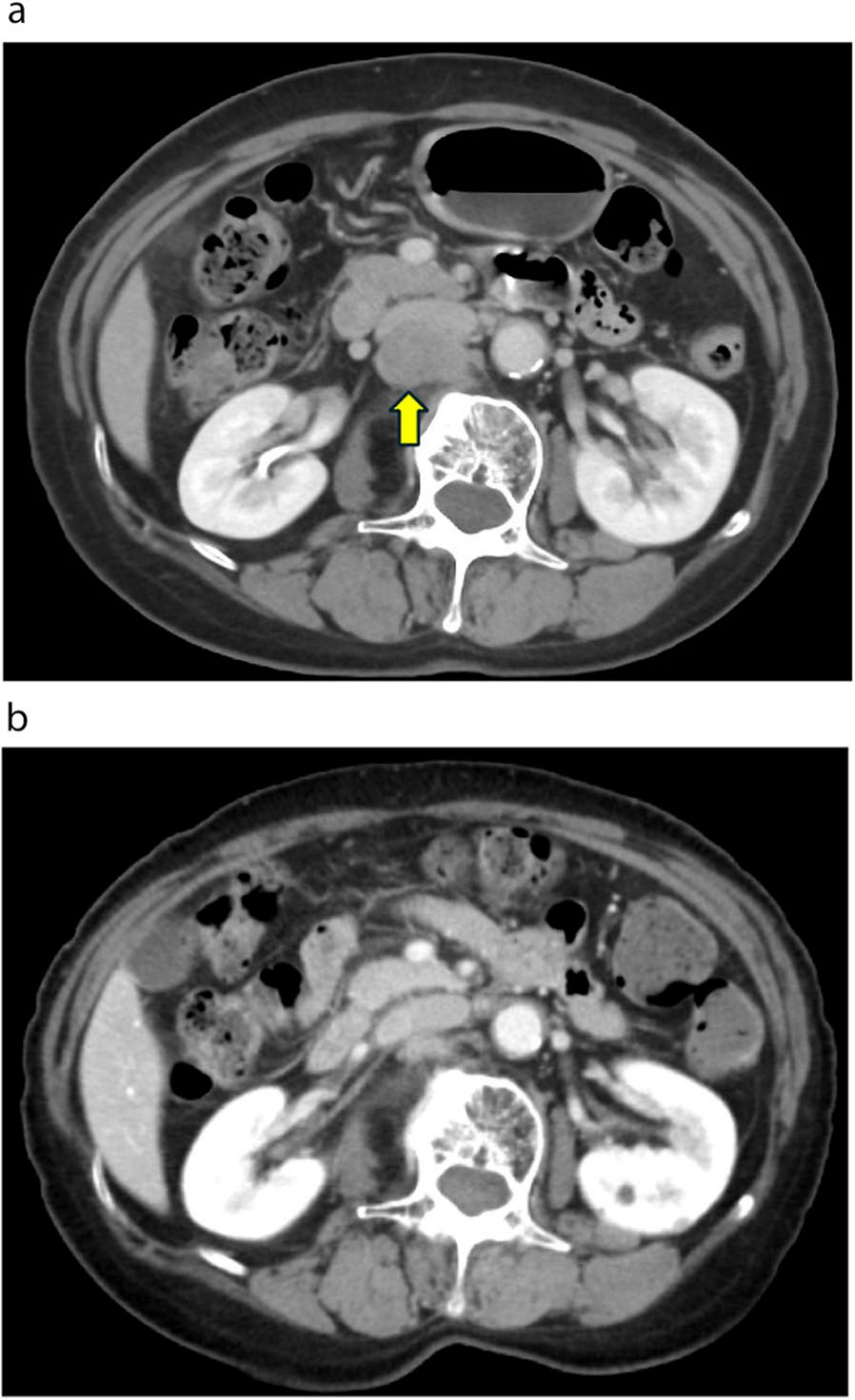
Para-aortic lymph node status pre- and post-radiation therapy assessed using axial CT imaging. a Para-aortic lymph node recurrence is observed again (arrow). b After intensity-modulated radiation therapy is initiated, para-aortic lymph node recurrence demonstrates CR. *CR* complete response, *CT* computed tomography
